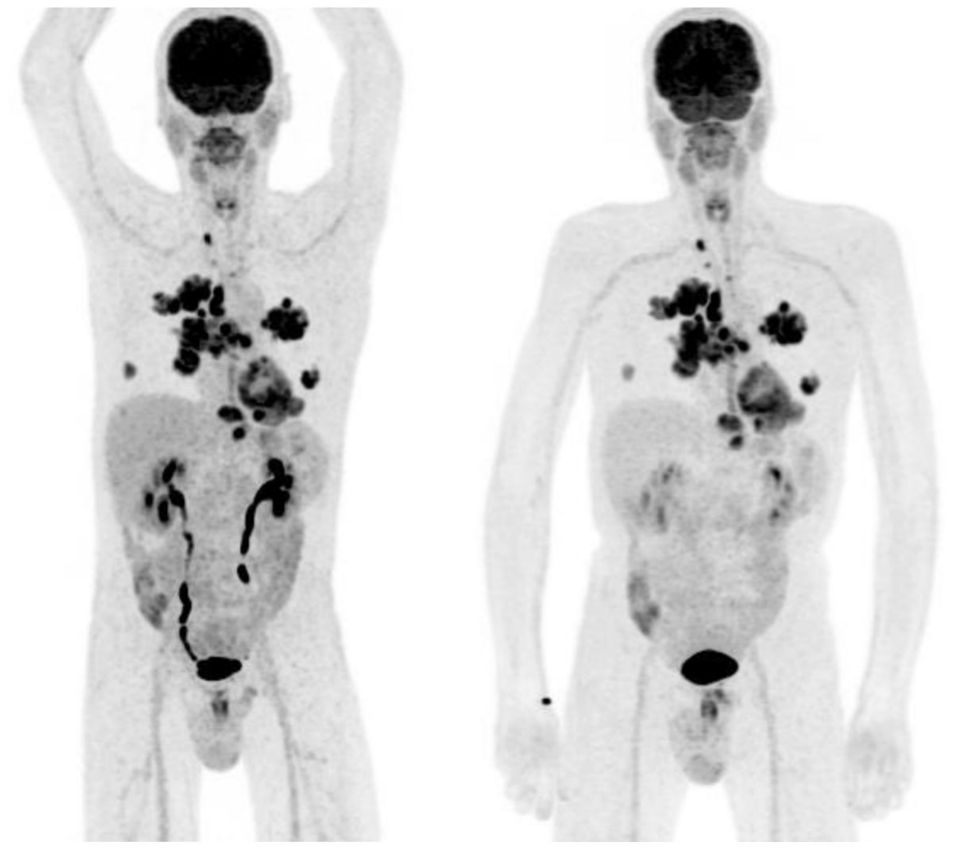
Three-dimensional maximum intensity projection of a PET/CT acquisition (left) and posterior PET/MR acquisition (right) of the same patient.
